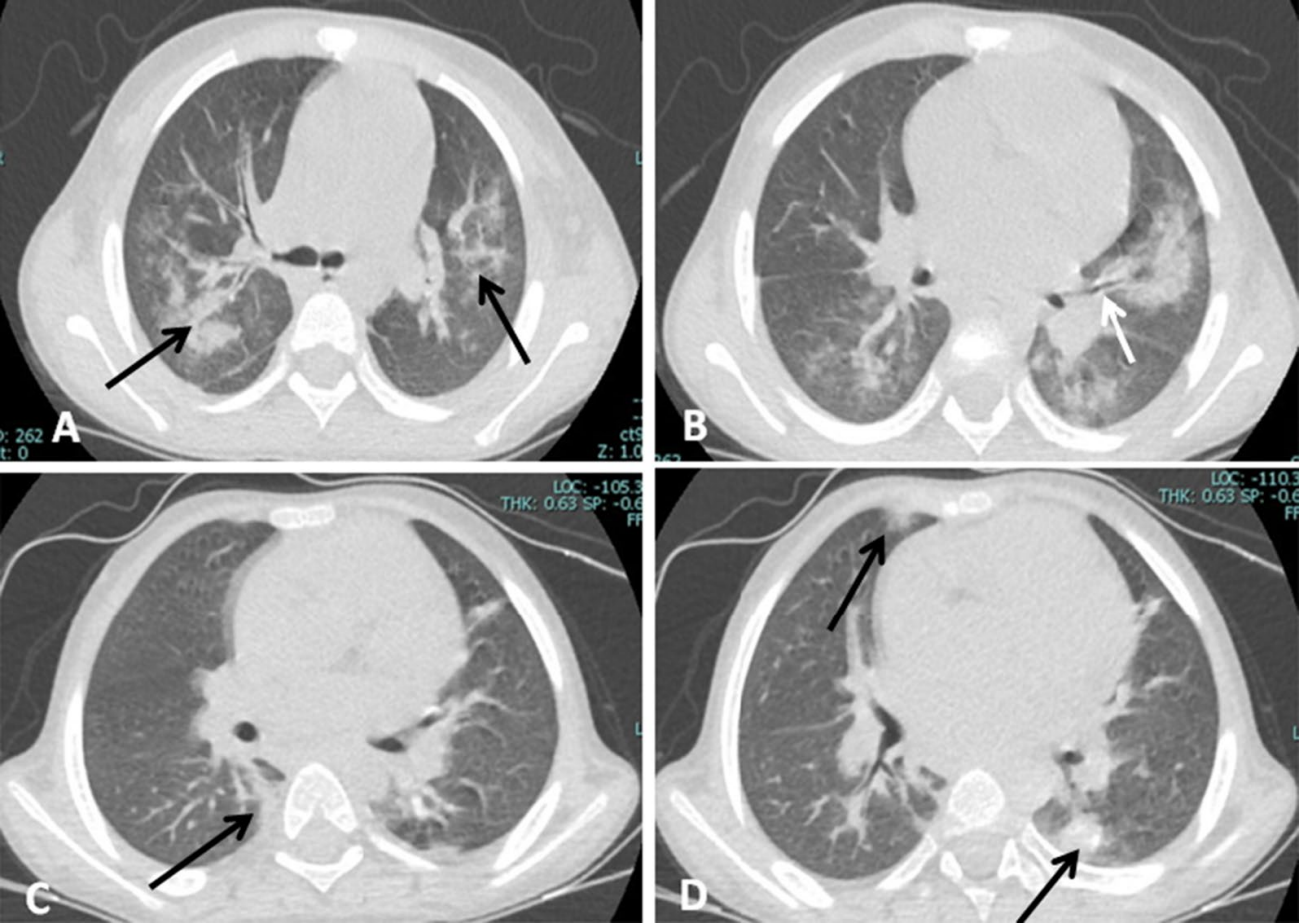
(A, B) A 7-year-old male patient known case of Glanzman disease presented with bleeding from the nose with mild respiratory manifestation and PCR positive for COVID-19. CT axial images, showing bilateral central consolidation with areas of centrilobular nodular infiltrates of air spaces (black arrows); findings are indeterminate for COVID-19, and CT severity score was 10/20. An air bronchogram is seen in the left lung (white arrow). (C, D) A 4-year-old male patient diagnosed with HLH presented with a fever. CT axial images, showing bilateral peripheral ground glass opacities and consolidations (black arrows); findings are typical for COVID-19, and CT severity score was 4/20. Cardiomegaly is also noted
